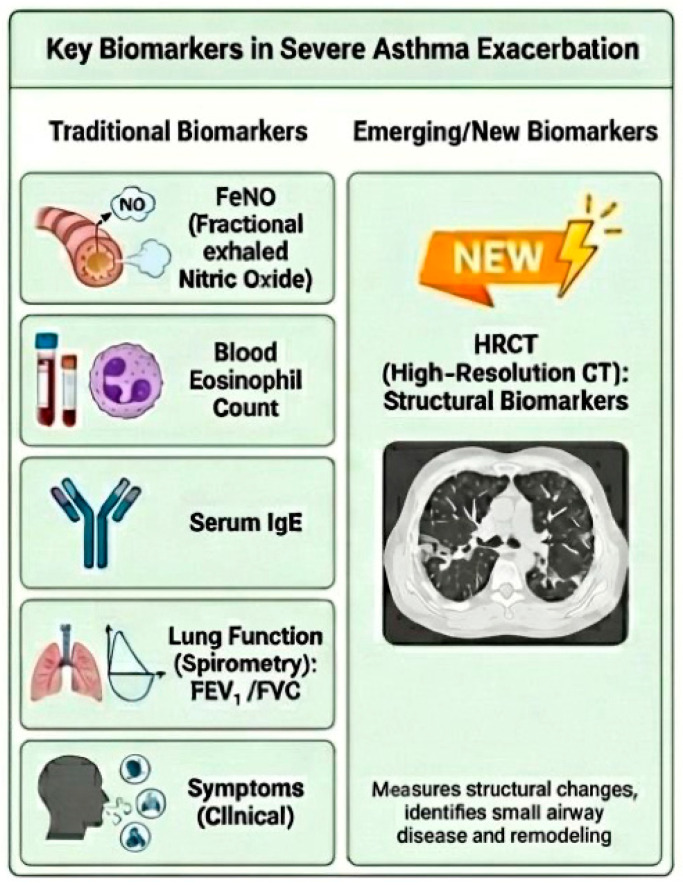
Key biomarkers in severe asthma exacerbation. NO: nitric oxide; FeNO: fractional exhaled nitric oxide; IgE: immunoglobulin E; FEV1: forced expiratory volume at first second; FVC: forced vital capacity; HRCT: high-resolution computed tomography.
